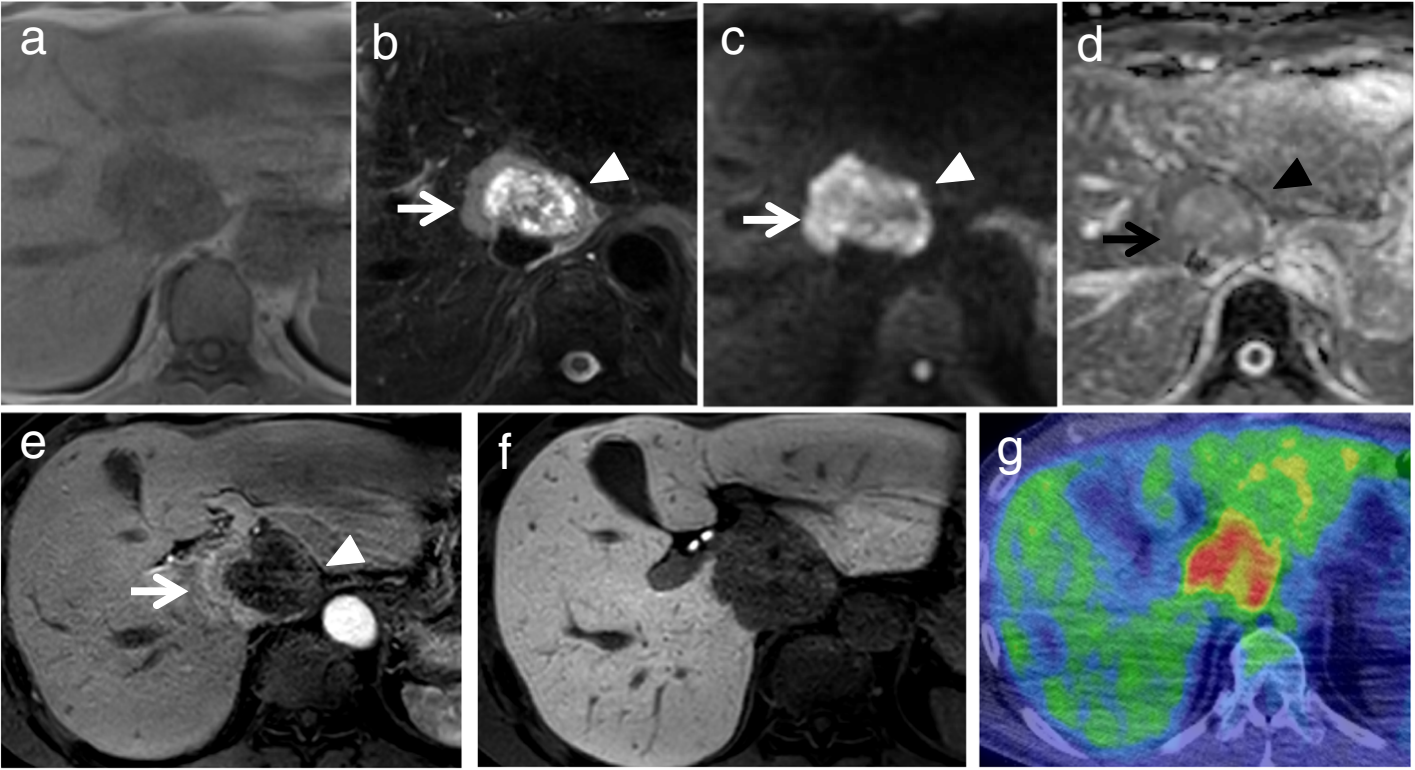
Magnetic response imaging (MRI) and [^18^F]-fluorodeoxyglucose position tomography (FDG-PET). MR images show both components with low intensity on T1-weighted images (a) and right component (arrow) of iso-high intensity and left component (arrowhead) of heterogeneously high on T2-weighted images (b). DWI showed higher intensity than normal liver parenchyma with a high *b* value of 1000 (c). Its ADC value was 1.19 × 10^− 3^ mm^2^/s (arrow on right) and 1.95 × 10^− 3^ mm^2^/s (arrowhead on left) (d). EOB-MRI showed right component (arrow) of the tumor as a hyperintense lesion but left component (arrowhead) as a hypointense lesion during the arterial phases (e) and hypointense mass in the hepatobiliary phase (f). FDG-PET shows accumulation of [^18^F]-FDG at both components (g)
